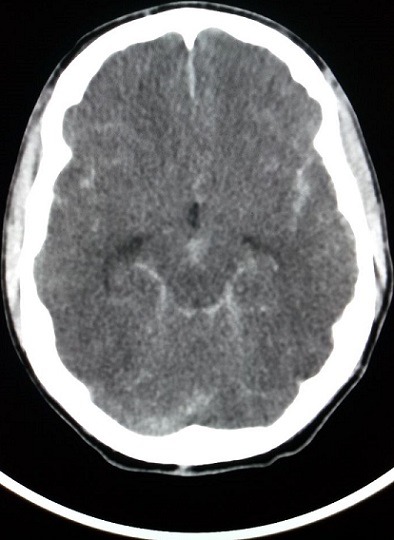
Unenhanced CT axial image demonstrates extensive subarachnoid haemorrhage with blood filling the central basal cisterns, bilateral Sylvian fissures and the anterior inter-hemispheric fissure with associated early hydrocephalus
